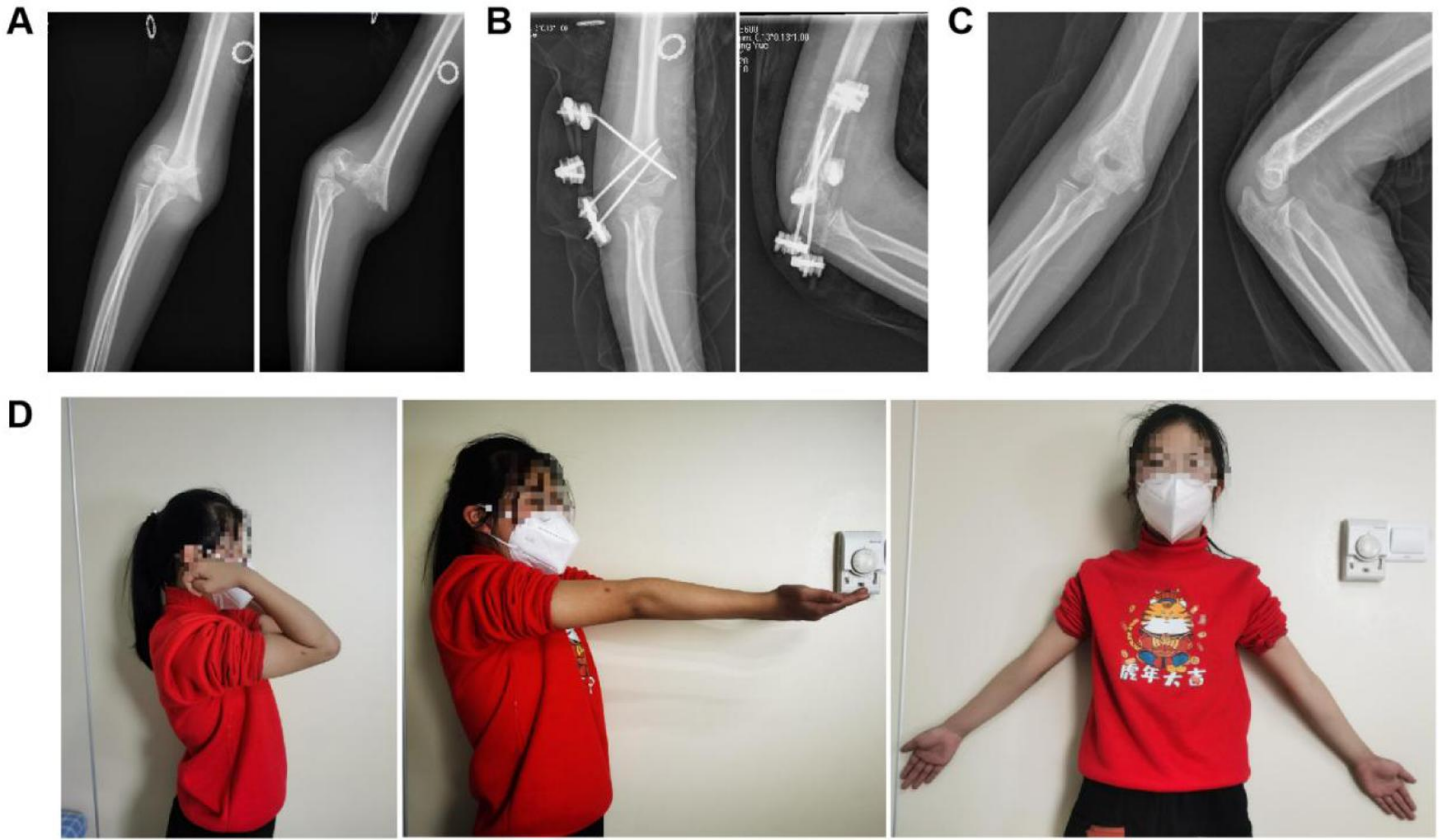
A case example of a 10-year-old girl with a right Gartland III supracondylar humerus fracture. (A) Preoperative radiographs, (B) 1-month postoperative radiographs, (C) 6-month postoperative radiographs, and (D) clinical photograph demonstrating symmetrical upper limb alignment.
